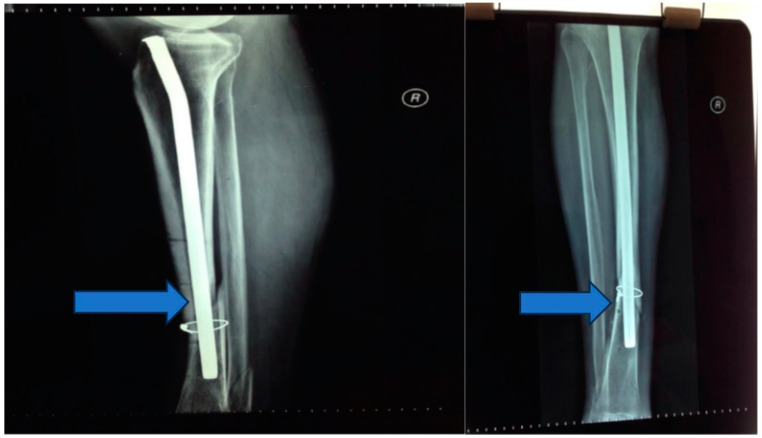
Postoperative X-ray—2 months.
